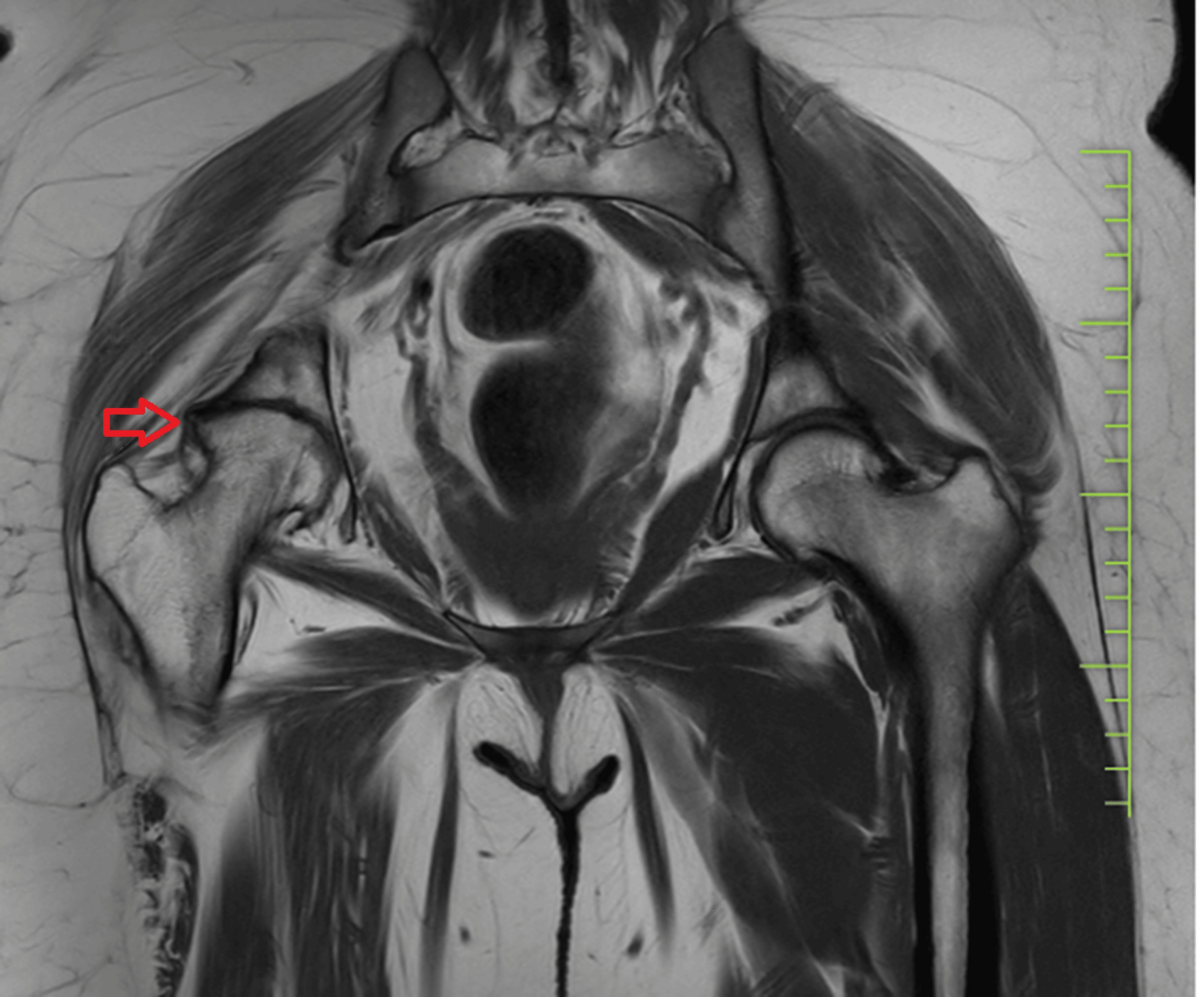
MRI of the pelvis with both hip coronal sections showing osteoarthritis of the right hip with reduced joint space and erosion of acetabular and femoral head surfaces. MRI: magnetic resonance imaging
